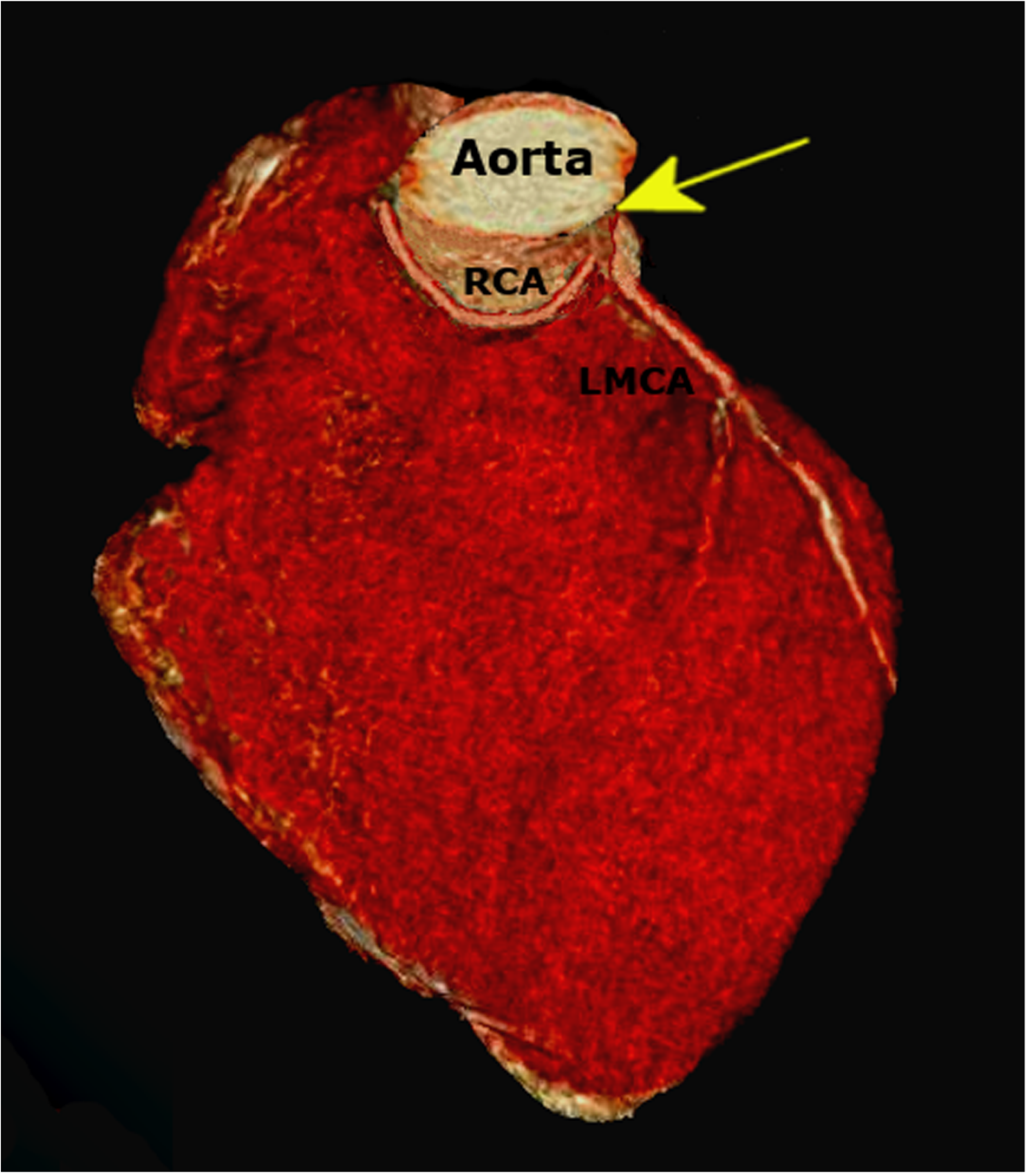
Computed tomography (CT)-based three-dimensional (3D) reconstruction showing the origin of RCA from the left sinus of Valsalva (arrow). The normal origin of the left main coronary artery (LMCA) is also seen
